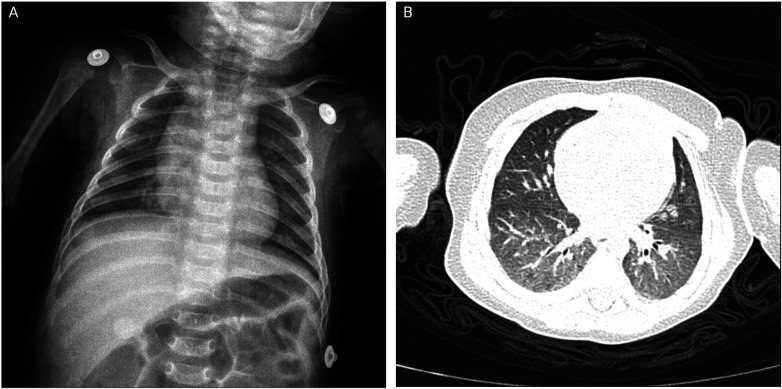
Radiological findings of the patient's respiratory infection. (A) Anteroposterior chest radiograph revealing diffuse, patchy infiltrates and increased lung markings bilaterally, suggestive of extensive bronchopneumonia. (B) Axial high-resolution computed tomography scan of the chest (lung window) demonstrating multifocal ground-glass opacities, patchy consolidations, and peribronchial thickening, consistent with a severe, multi-lobar pulmonary infection.
